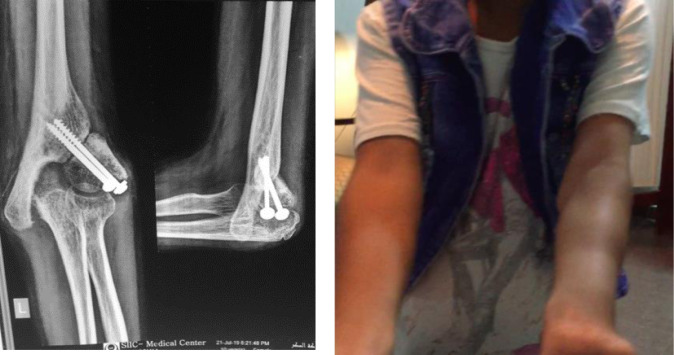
Early postoperative X-ray and 6-month postoperative clinical photo of case no. (1) (left elbow).
